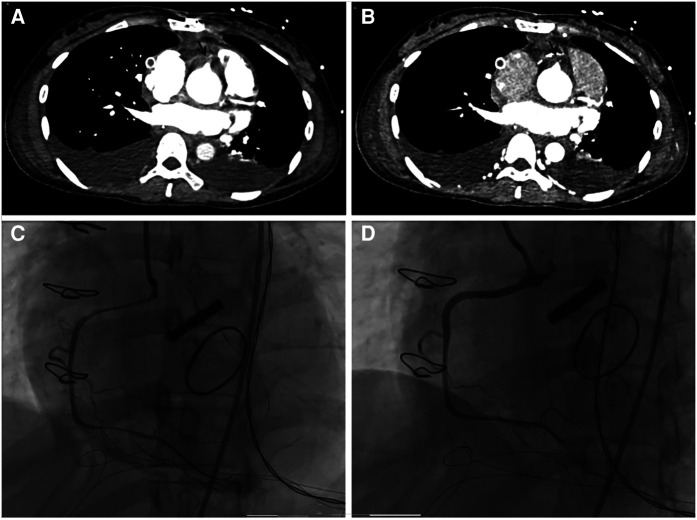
(A,B) pulmonary artery computed tomography angiography showed occlusion of the initial of the right coronary artery (black arrow). (C) Coronary angiography revealed occlusion of the right coronary artery (RCA) ostium, which was significantly improved after percutaneous coronary intervention (D).
